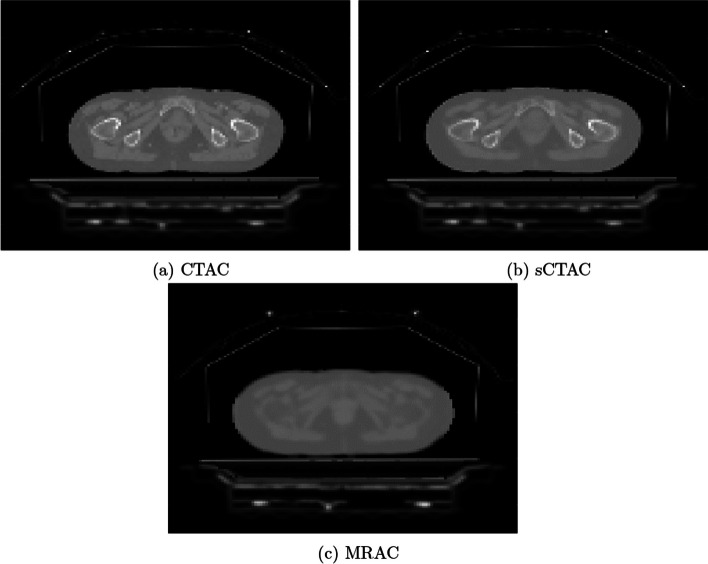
Attenuation correction maps for an example patient. All maps included the MR coils components, flat couch top and coil bridge. Patient models were based on CT (a), ZTE-derived sCT (b) and Dixon derived MR (c)
